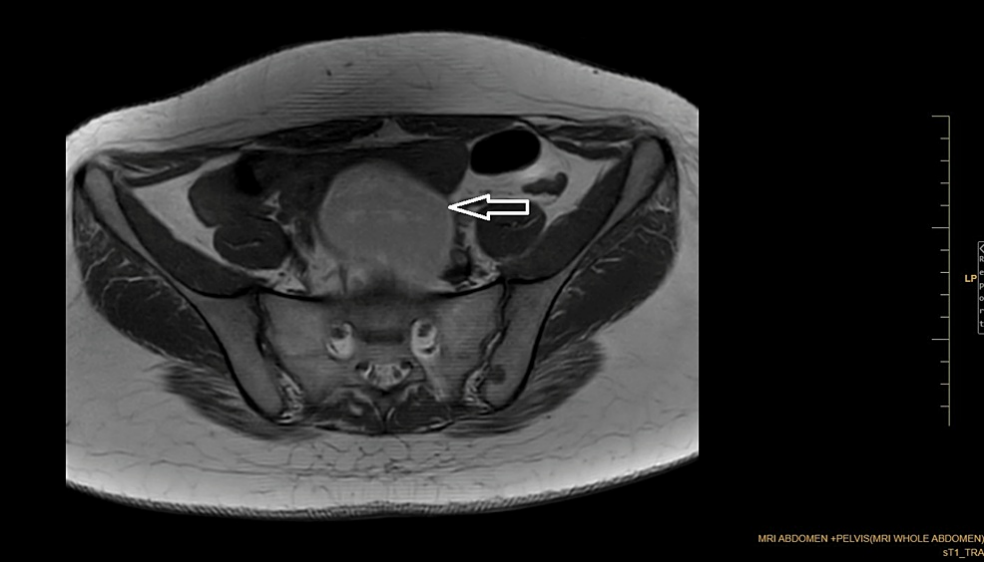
Axial T1WI showing hyperintense collection in the vagina suggestive of hematocolpos. Note: Authors' original image T1W1: T1-weighted image
